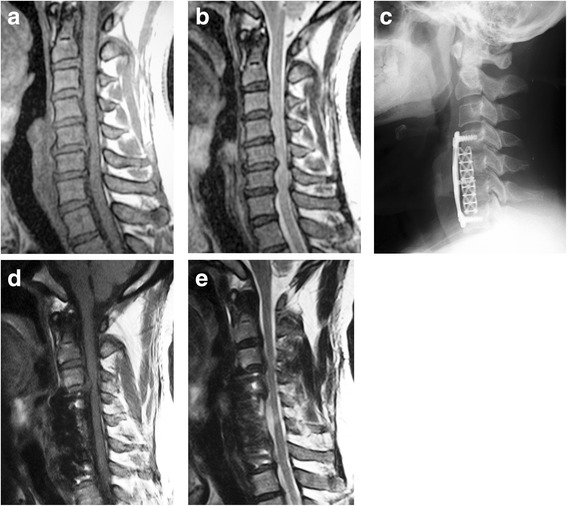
47-year-old man with multilevel cervical intervertebral disc herniation. a, b Preoperative T1-weighted and T2-weighted MRI shows an indentation of dura mater at C3-C4, C4-C5, C5-C6 and C6-C7 levels. c Radiograph after operation shows 3-level fusion from C4-C7. d, e MRI at 6 years after operation indicates complete decompression at C4-C5, C5-C6, and C6-C7 levels, but C3-C4 level shows significant spinal cord compression
